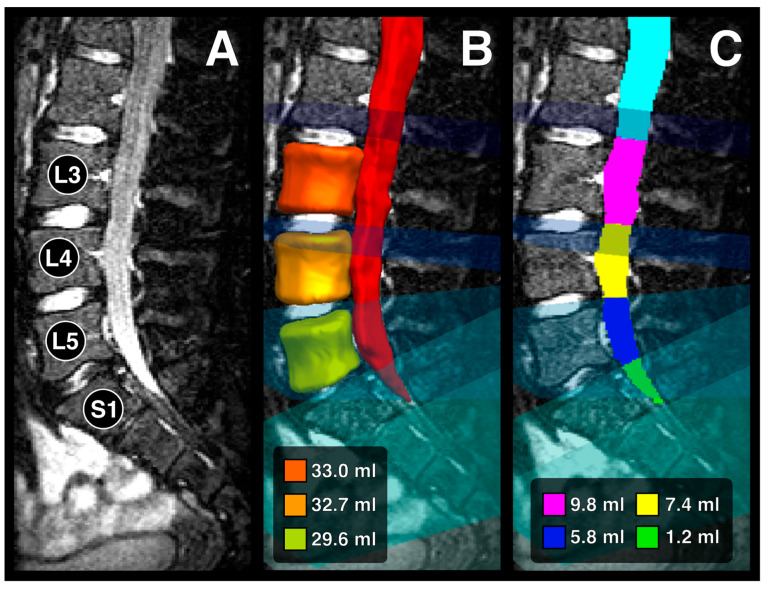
Representative example of a 3D segmentation of the lower lumbar vertebrae and dural sac segments in a non-MFS patient. (A) Sagittal view of the lumbosacral MRI. (B) Visualization of the 3D segmentation and volumes of the vertebrae. (C) Visualization of the intervertebral clipping planes and the resulting dural sac segments, including their volumes.
